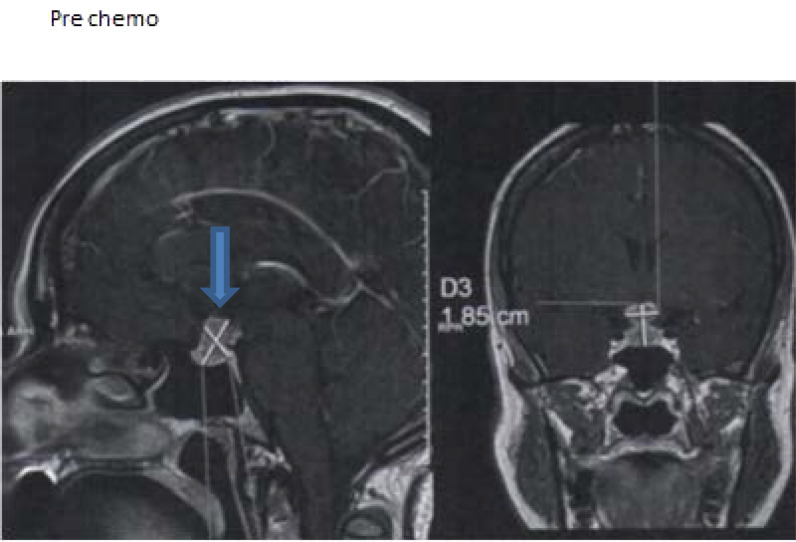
Case 3. Magnetic resonance image of the pituitary gland before chemotherapy.
